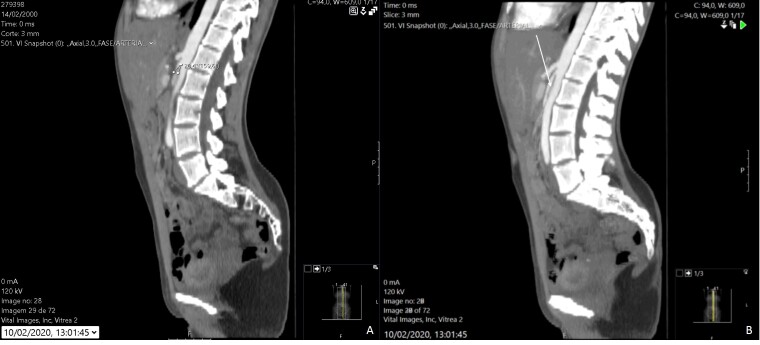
Saggital plane of an abdominal CT showing a decreased aortomesenteric angle (A), causing compression of the left renal vein (white arrow) (B).
